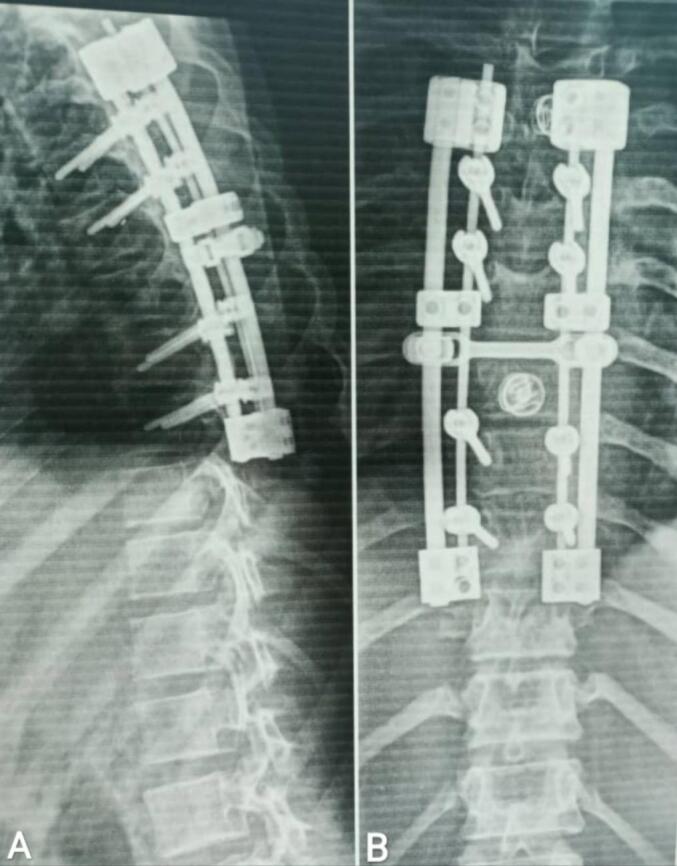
Anteroposterior (image B) and lateral (image A) X-rays of the thoracic spine reveal a transpedicular fixation device comprising eight pedicle screws at T5, T6, T8, and T9 levels, along with connectors and four rods. Lateral mass screws were utilized due to the unavailability of pediatric devices.
